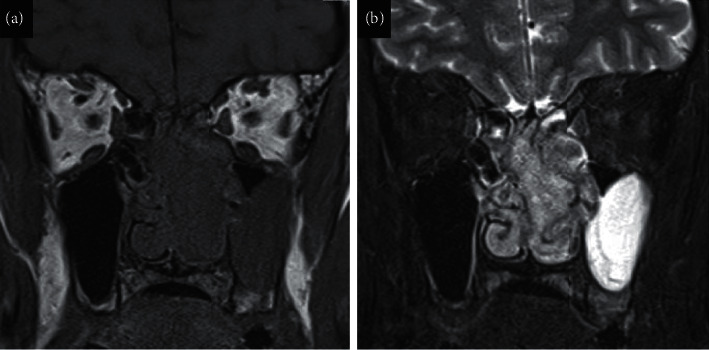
Magnetic resonance imaging. (a) T1-weighted image on coronal section. (b) T2-weighted image on coronal section.
